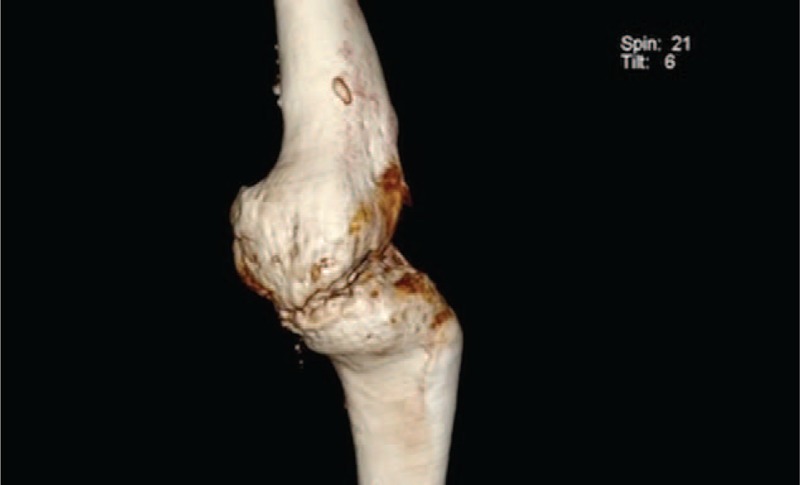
The computed tomography three-dimensional scan with signs of bone fusion.
